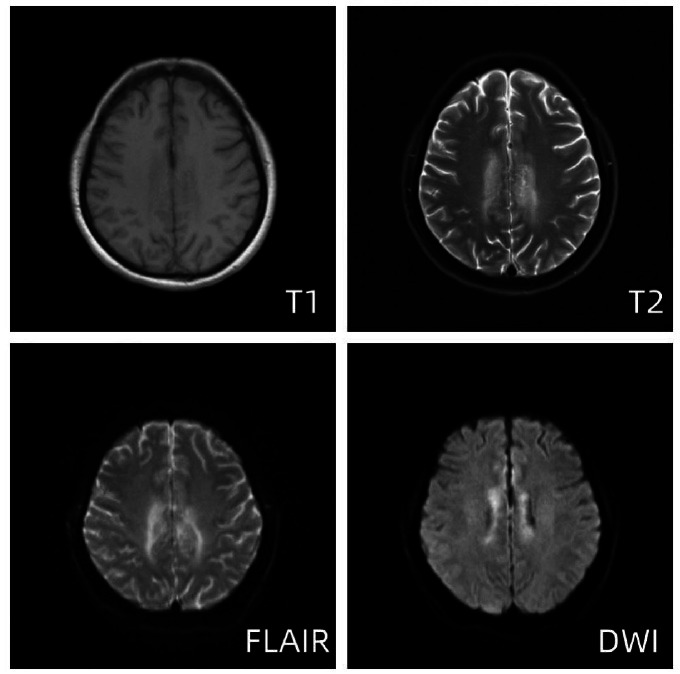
MRI of the brain on day 20 after onset of the disease. MRI results indicated slightly decreased signal intensity on T1-weighted images, with small bands of high signal observed near the midline of the bilateral frontoparietal lobes (specifically in the paracentral lobule region) on T2 and FLAIR sequences. DWI revealed restricted diffusion areas in bands adjacent to the midline of the bilateral frontoparietal lobes, showing a notable reduction in size compared to previous scans. The remaining brain parenchyma did not exhibit any conspicuous abnormal signal patterns.
